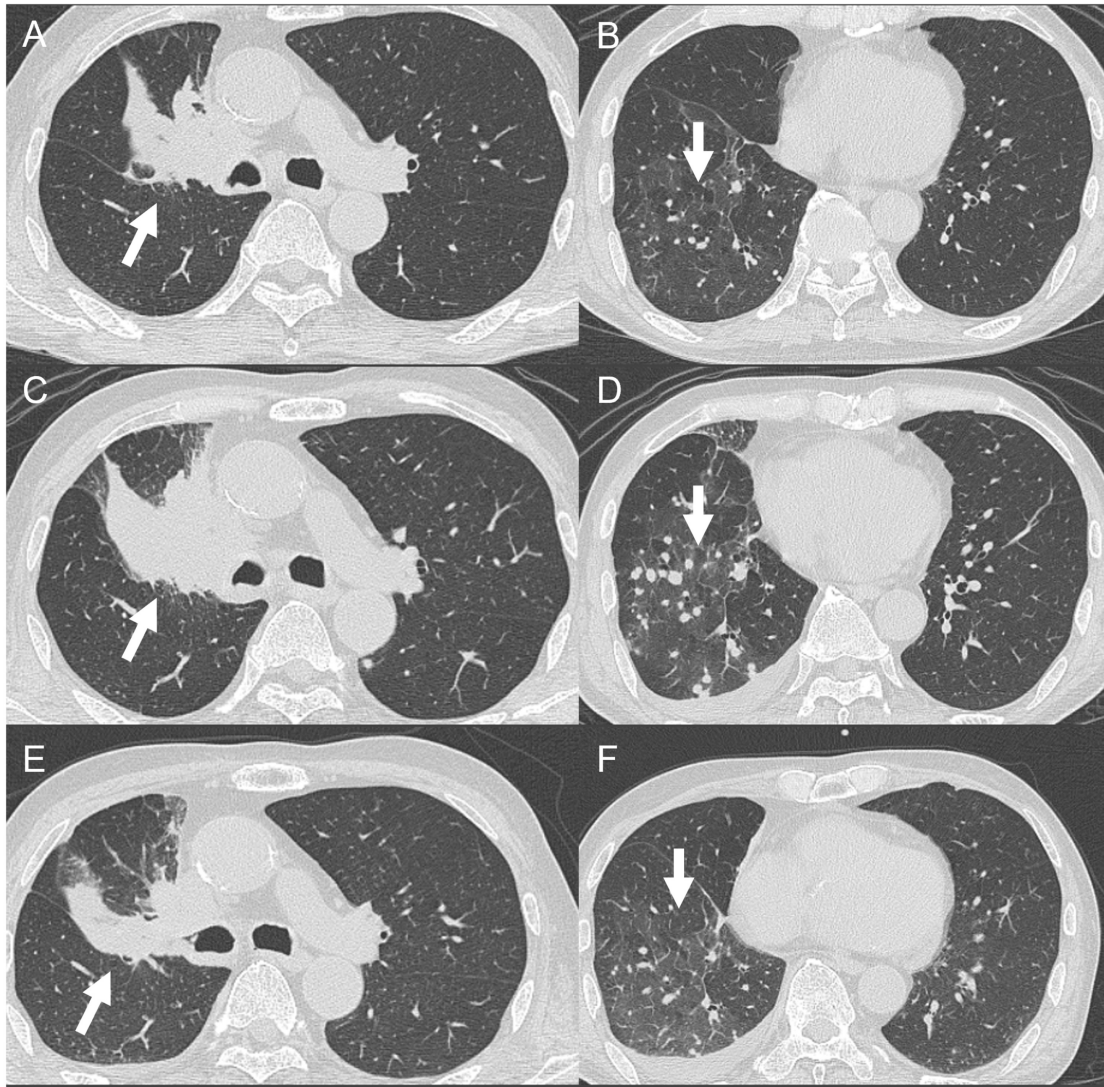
Radiographic course on chest CT scan. Right hilar mass and right lower lobe metastases enlarged transiently on day two (C, D) and subsequently decreased in size on day seven (E, F). Arrows indicate the areas of interest. (A) CT image at the carinal level on day -four. (B) CT image of the lower lobe level on day -four. (C) CT image at the carinal level on day two. (D) CT image at the lower lobe level on day two. (E) CT image at the carinal level on day seven. (F) CT image at the lower lobe level on day seven.
